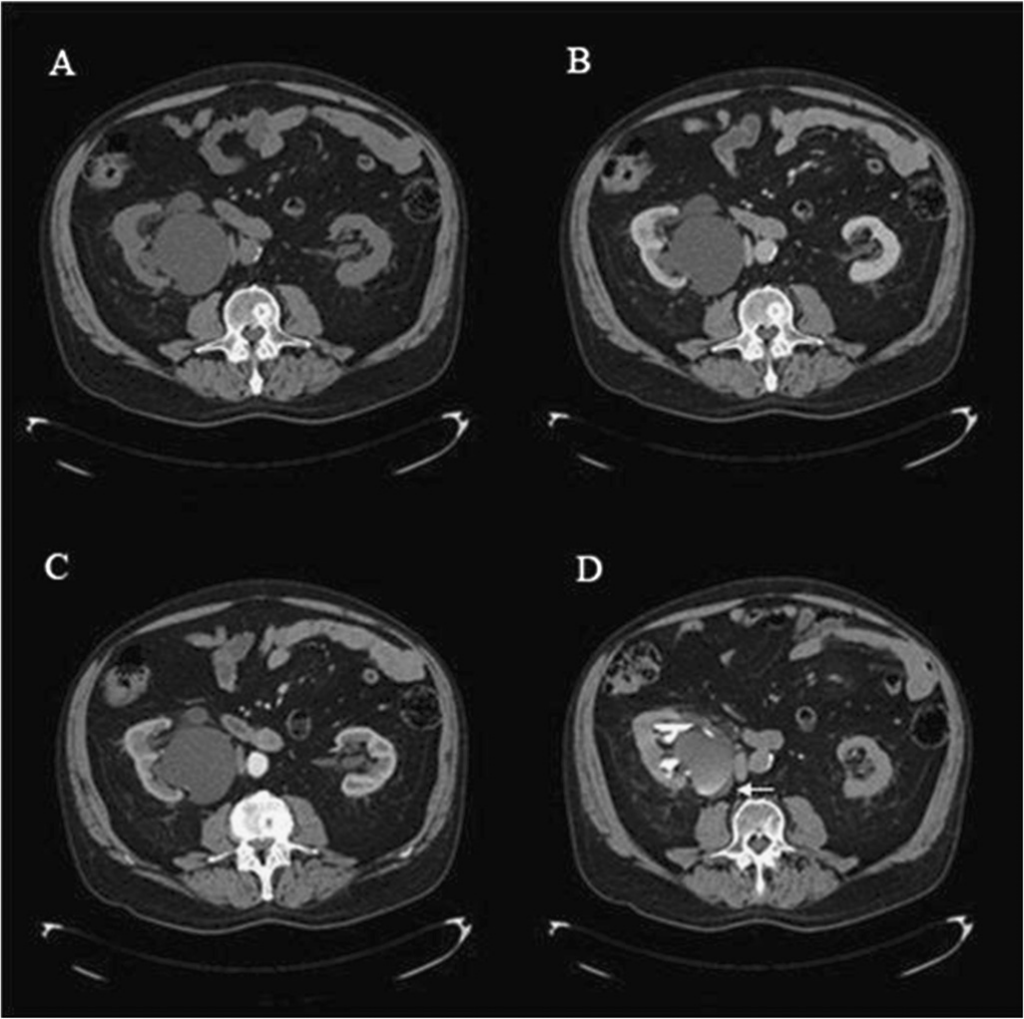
Pre-contrast (A), venous (B), arterial (C) and excretory (D) phases of the current computed tomography scan. Note the evolution of the cyst during the acquisition of the multiphase computed tomography images. The excretory phase (D) demonstrates that the cyst is smaller than the other phases (A, B and C), there is contrast inside and free fluid around (white arrow), also not present on the previous aquisitions.
